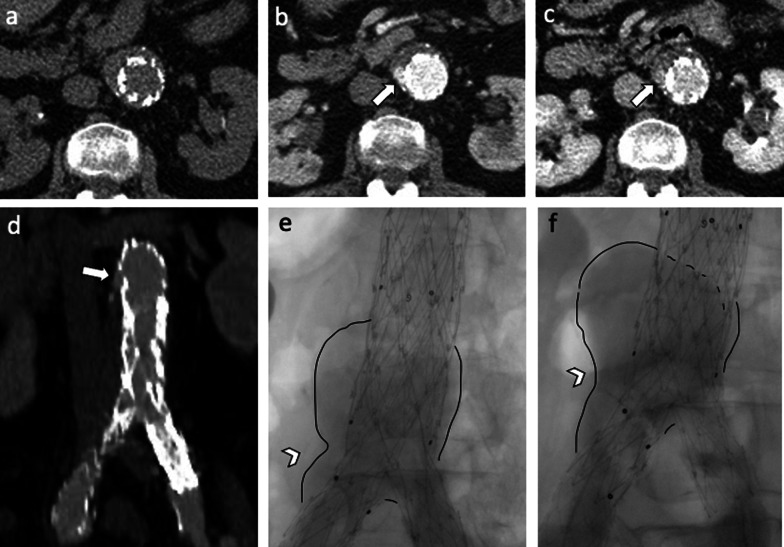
67-year-old woman with *type IIIb* endoleak: Axial CT images on (a) pre-contrast, (b) arterial and (c) delayed phases and (d) coronal arterial phase show a type IIIb endoleak (arrows); intraoperative aortography in (e) anteroposterior and (f) caudo-cranial projections performed in the same patient confirmed endoleak (arrowheads) with profile remarked through a black line
